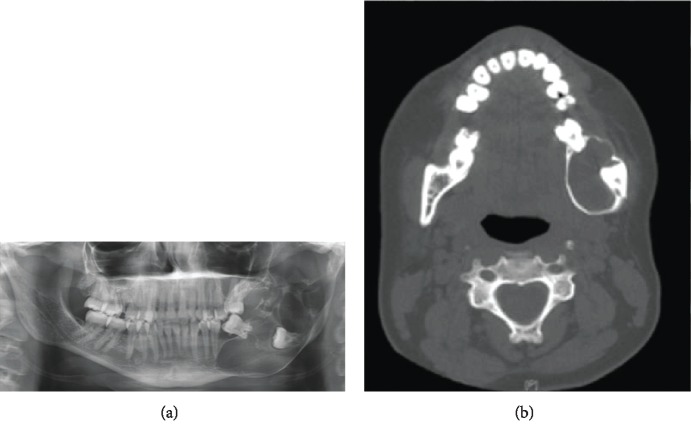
(a) Orthopantomogram showing an impacted 38 with an associated multilocular radiolucent lesion involving the left posterior mandible, which extends from the second premolar to the left condyle. (b) Axial view of a computed tomographic image of the facial bone showing a hypodense lesion in the left posterior mandible, with expansion and thinning of both the buccal and lingual cortices.
